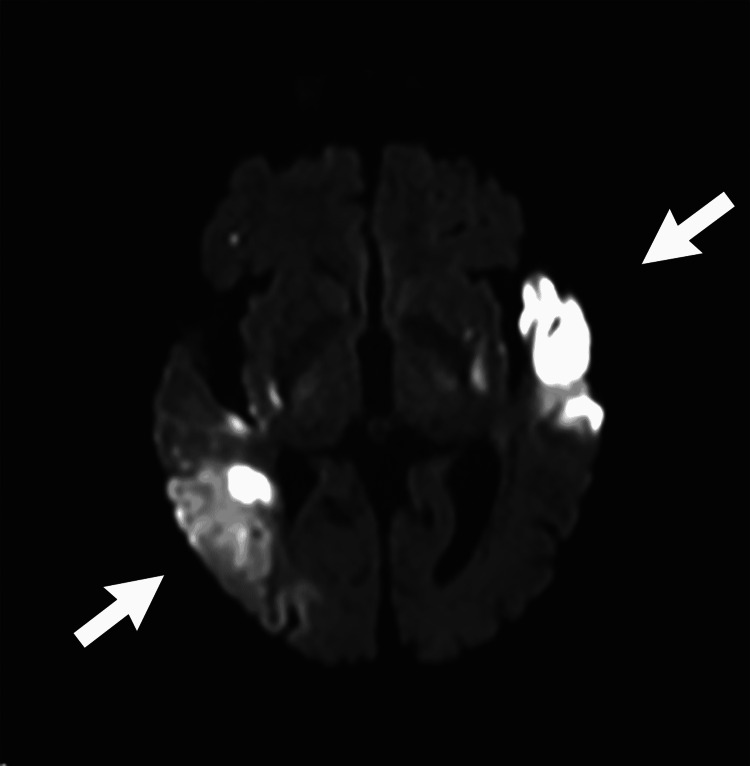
MRI showed areas of diffusion restriction in the left anterior temporal lobe, left basal ganglia, and right posterior temporoparietal region
